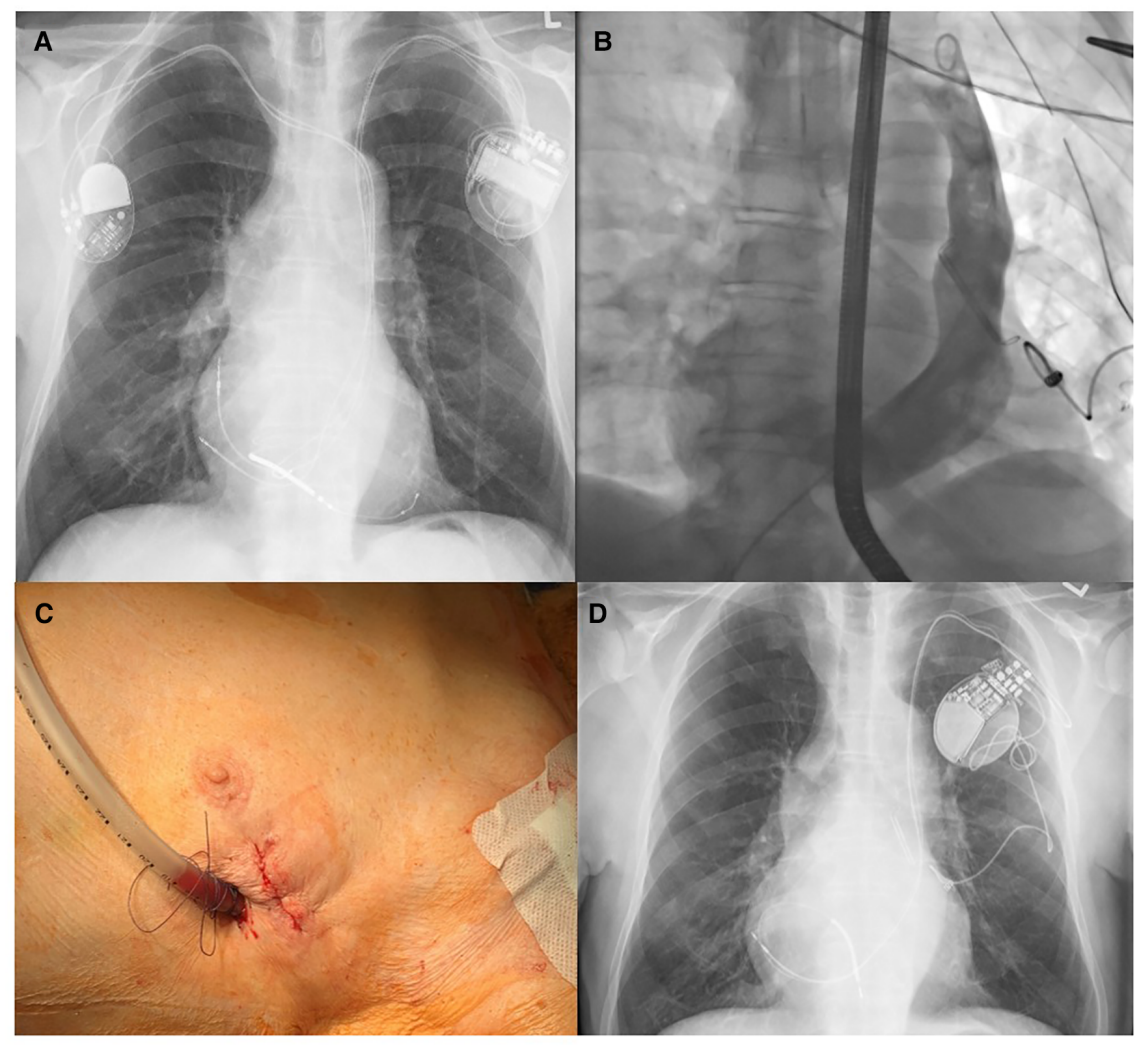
(A) Preoperative chest x-ray showing the left-sided CRT-D device and the right-sided pacemaker through left-sided superior vena cava (SVC). (B) Intraoperative venography, after extraction of all leads, displaying the left-sided SVC and the epicardial LV-lead as well as the AtriClip®. (C) Minimally invasive left-lateral incision in the fourth intercostal space after wound closure. (D) Postoperative chest x-ray showing the AtriClip® and the LV lead connected to a single-chamber pacemaker.
